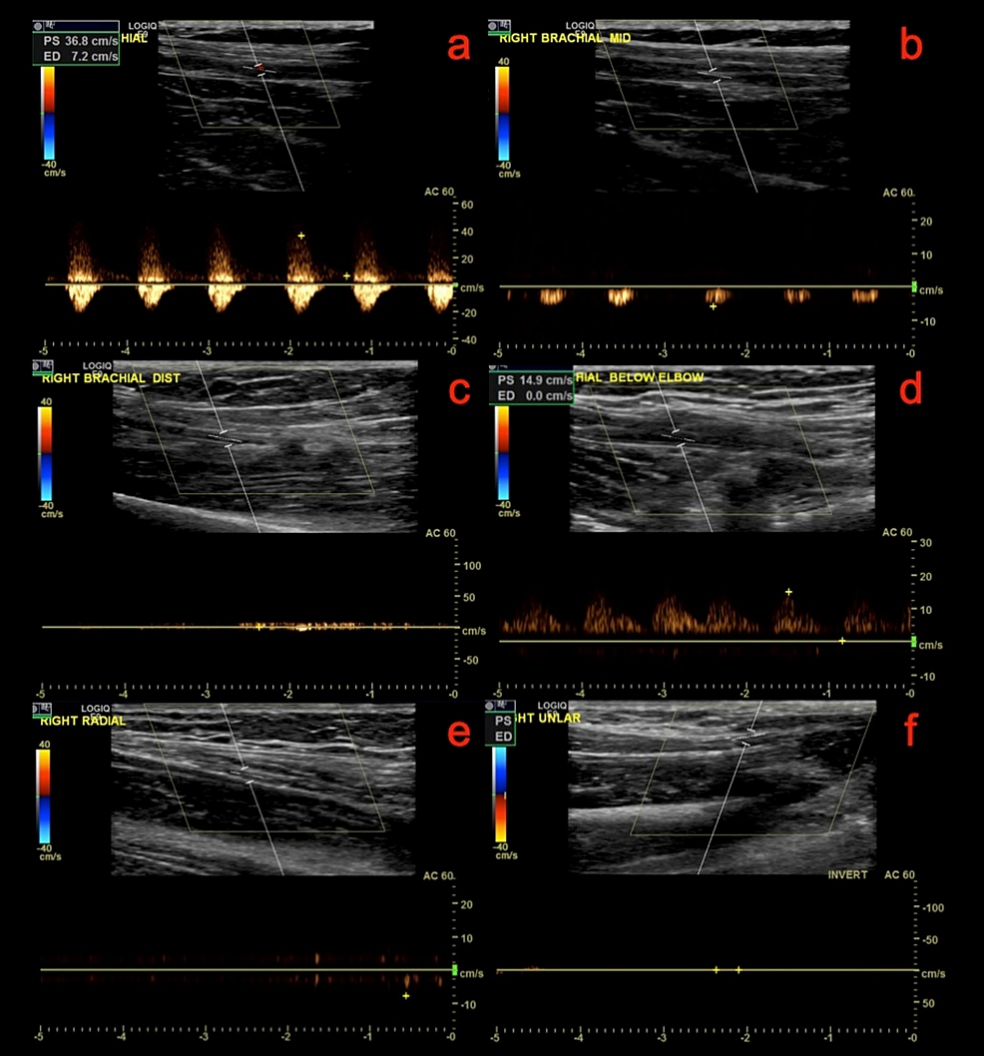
Arterial Duplex Ultrasound evaluation at different locations: a) right brachial proximal; b) right brachial mid; c) right brachial distal; d) right brachial distal below the elbow; e) right radial; f) right ulnar
